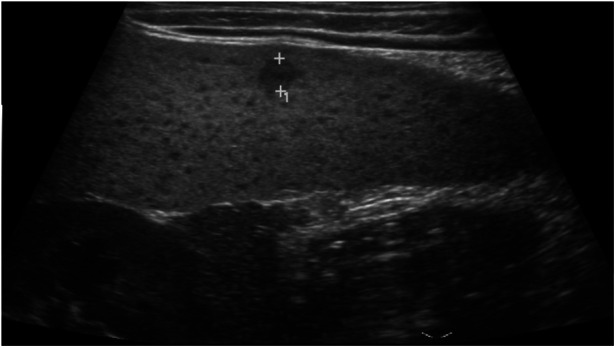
Image of an 8-year-old girl with a skin rash, prolonged fever, and multiple small splenic abscesses who was diagnosed with childhood-onset SLE. Abdominal sonogram showing a 0.5 cm hypoechoic splenic lesion.
